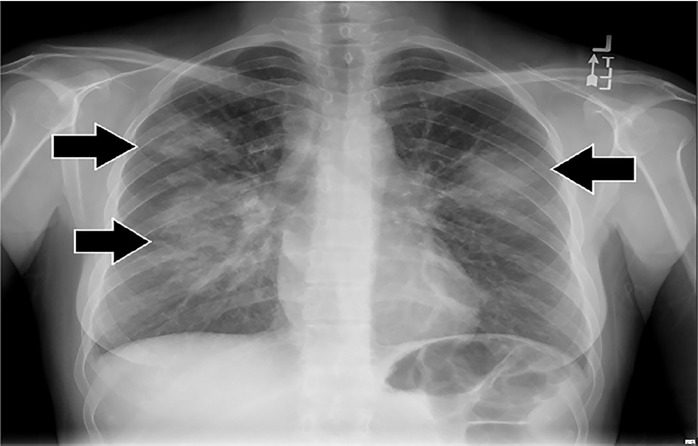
Chest radiograph of a 38-year-old patient with rash and dyspnea, showing bilateral multi-lobar infiltrates (arrows).
